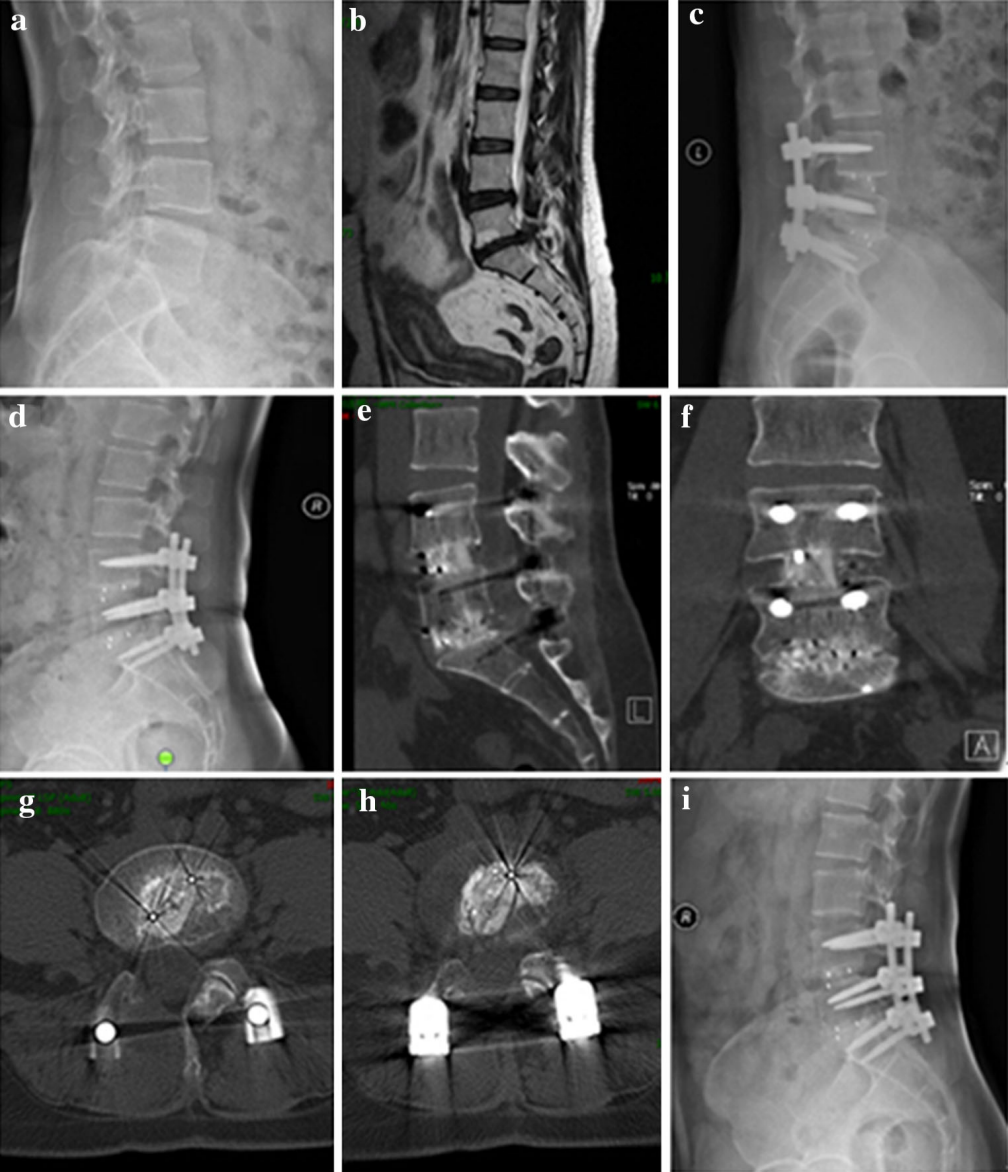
A 42-year-old female who underwent 2-level TLIF with n-HA/PA66 cages for lumbar reconstruction. The preoperative lumbar radiographs (a, b). The 1-week postoperative and 3-month follow-up radiographs (c, d). The CT or 3D-CT scan (e, f, g, h) shows that the autogenous bone granules fill the cages and achieve bony fusion with adjacent endplates by the 10-month follow-up. A lateral radiograph (i) at the final follow-up shows satisfactory bony fusion and no obvious migration, radiolucent gap or subsidence
